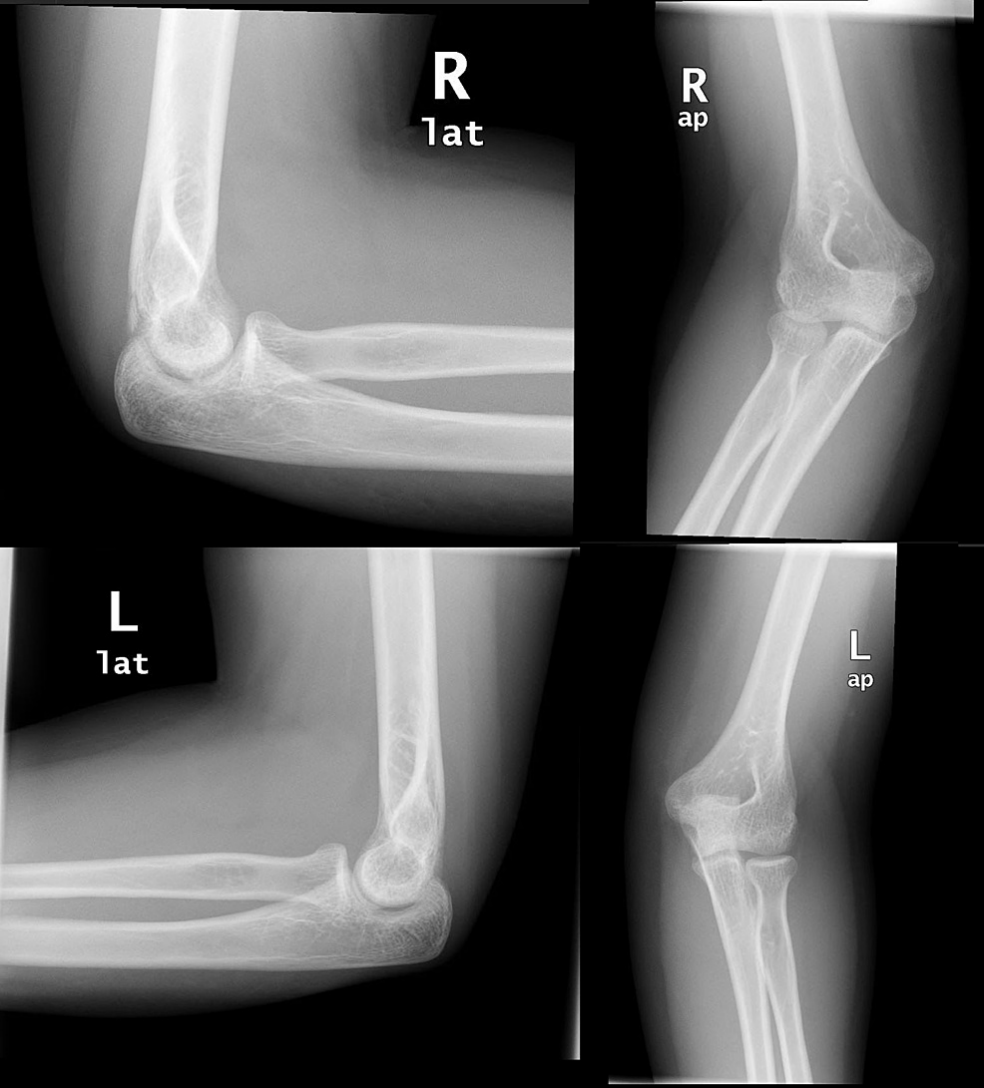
Post-reduction X-rays showing adequate reduction bilaterally.
